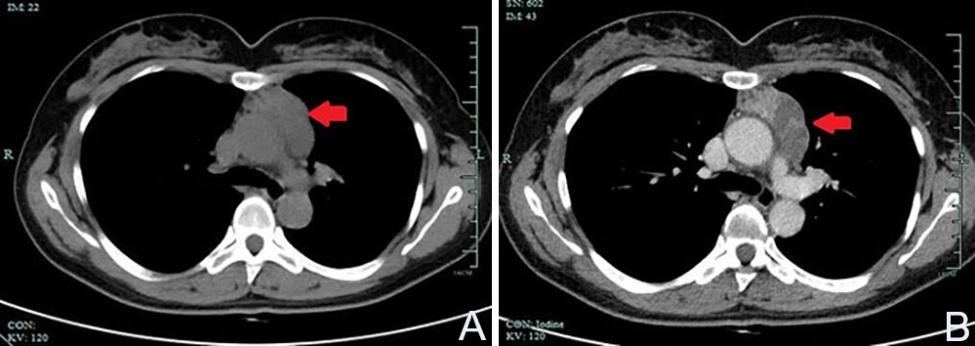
Chest-computed tomography revealed a cystic solid mass in the anterior mediastinum (A). Well-defined boundaries and mild to moderate heterogeneous enhancement of the solid portion and internal septum were detected by computed tomography-enhanced scans (B)
